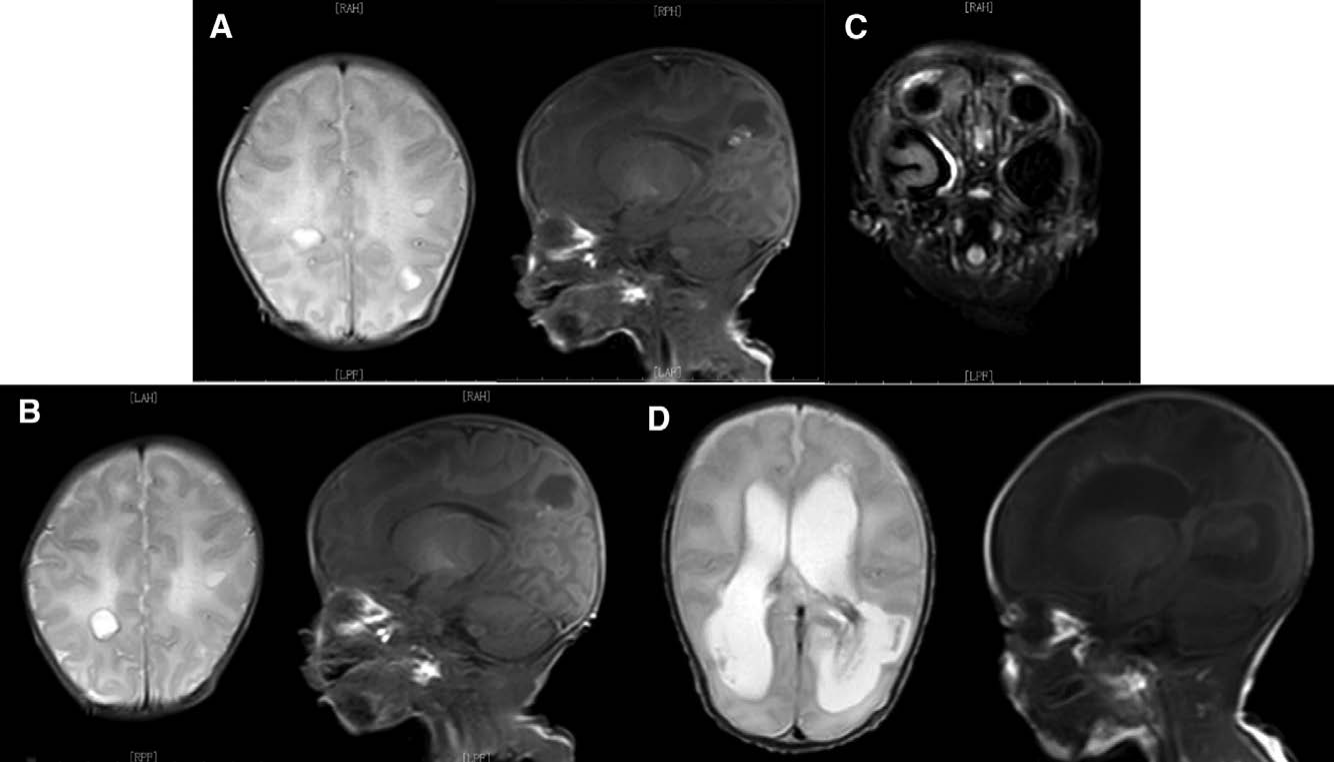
Cerebral MRI. (A and B) Hemorrhagic liquefactive necrosis of left hemisphere. (A) Abnormal hyperintensity at frontoparietal temporal lobes on both sides; T1WI sagittal view mixed low point hyperintensity, and hyperintensity on T2WI. (B) Reexamination after 2-wk treatment. Multiple patchy abnormal hyperintense foci at frontoparietal temporal lobes on both sides, with similar range to that in A; spotted hyperintensity on both T1WI and hypointensity on T2WI; while there was no significant change where T2WI had hyperintensity and T1WI had hypointensity (softening lesions). (C) T2-flair enhancement. Right temporal meningeal enhancement. Obvious enhancement (about 2.8 cm) was observed at the right temporal meninx. (D) Encephalocele and parietal lobe on both sides showed platy low T1 signal and high T2 signal, with ill-defined borders, partially connected to the rear corners of encephaloceles on both sides; encephaloceles on both sides were enlarged, while diocoel and epicotyl had no obvious enlargement. MRI = magnetic resonance image.
